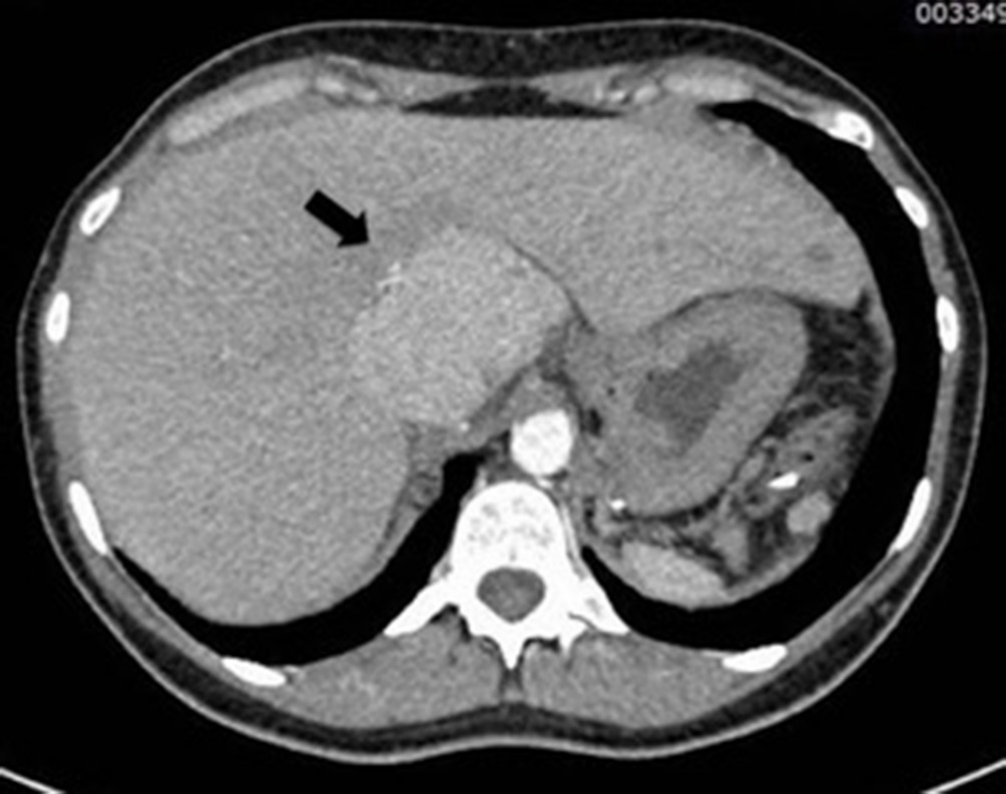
Axial CT scan shows a further contrast enhancing lesion in the liver in keeping with hepatic splenosis (arrow).
